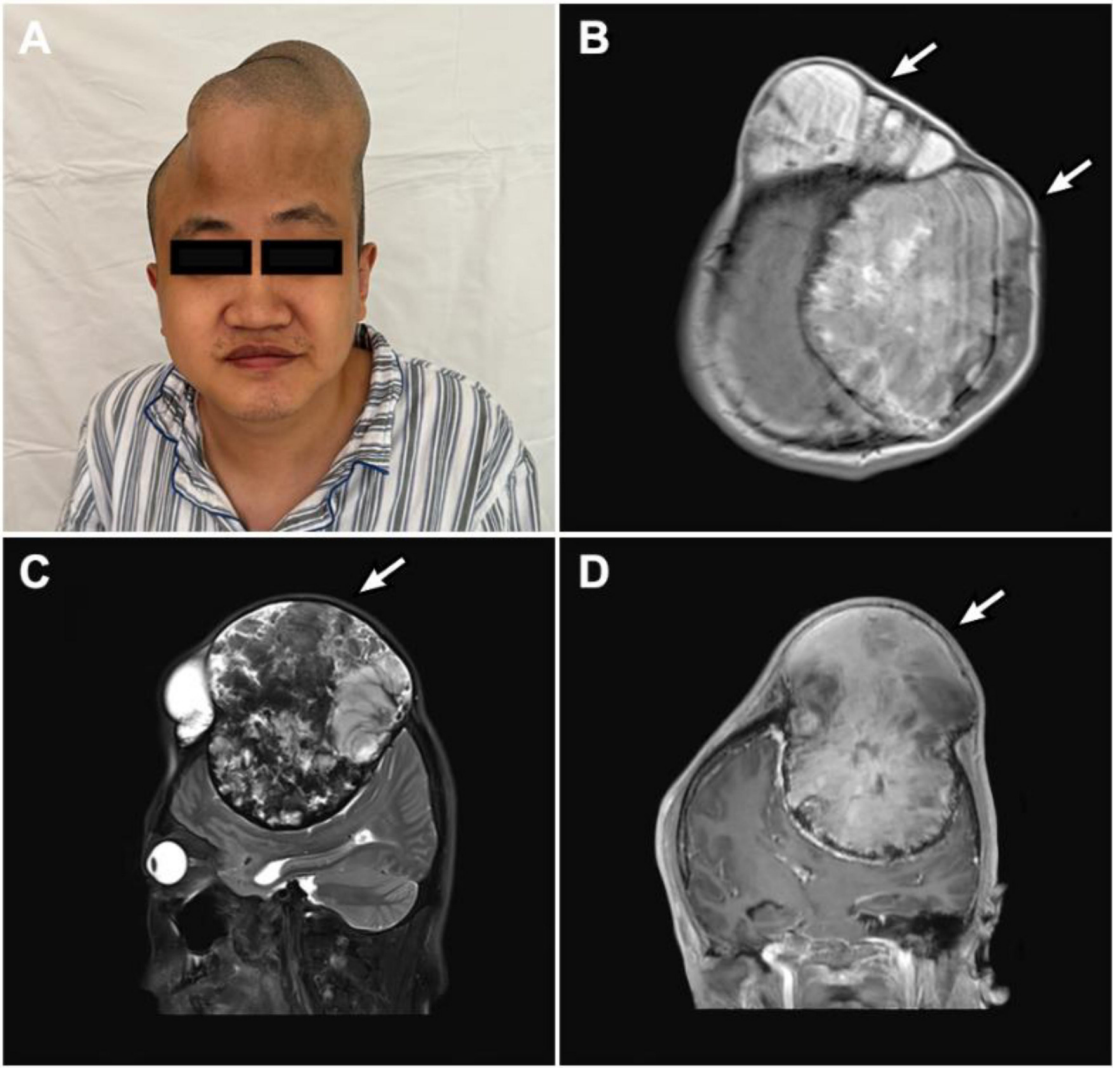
Clinical and radiological features. (A) Clinical photograph showing a large, firm cranial bulge over the left frontoparietal region; (B) axial T1-weighted MRI demonstrating a massive heterogeneous lesion with skull invasion and midline shift; (C) T2-weighted sagittal MRI showing craniocaudal tumor expansion and extracranial extension; (D) gadolinium-enhanced coronal MRI depicting the “mushroom-like” morphology and bony remodeling.
